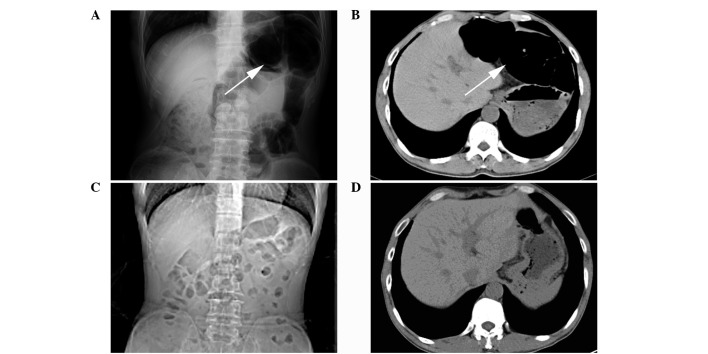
Radiological observations. (A) Plain abdominal X-ray revealed a gas shadow with visible haustral folds in the left subphrenic space (indicated by the arrow) prior to treatment. (B) Computed tomography (CT) identified an interposition of the left colon between the liver and the left diaphragm (indicated by the arrow) prior to treatment. (C and D) The gas shadow in the left subphrenic space and the colonic interposition in the CT scan disappeared following conservative treatment for one week.
